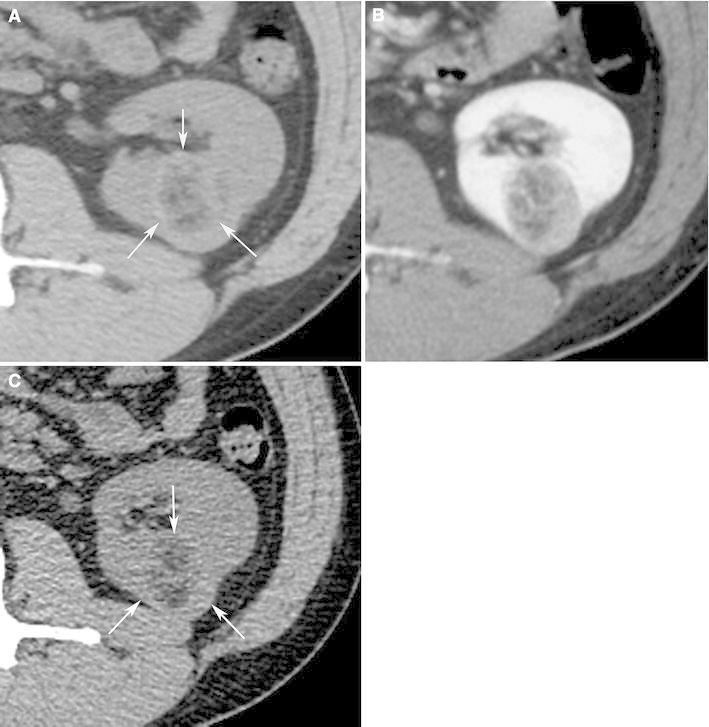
Angiomyolipoma, classic type, with small amount of fat in a 40-year-old man. Transverse, unenhanced CT (A) and enhanced (B) images (both using 5-mm sections) shows a 3.4-cm left renal mass (*arrows*) attenuation values that were measured on unenhanced images were all higher than −10 HU. When 1.5-mm sections were reconstructed (C), an attenuation of −25 HU was obtained and the diagnosis of angiomyolipoma was made. Fat attenuation was identified only on unenhanced CT.
